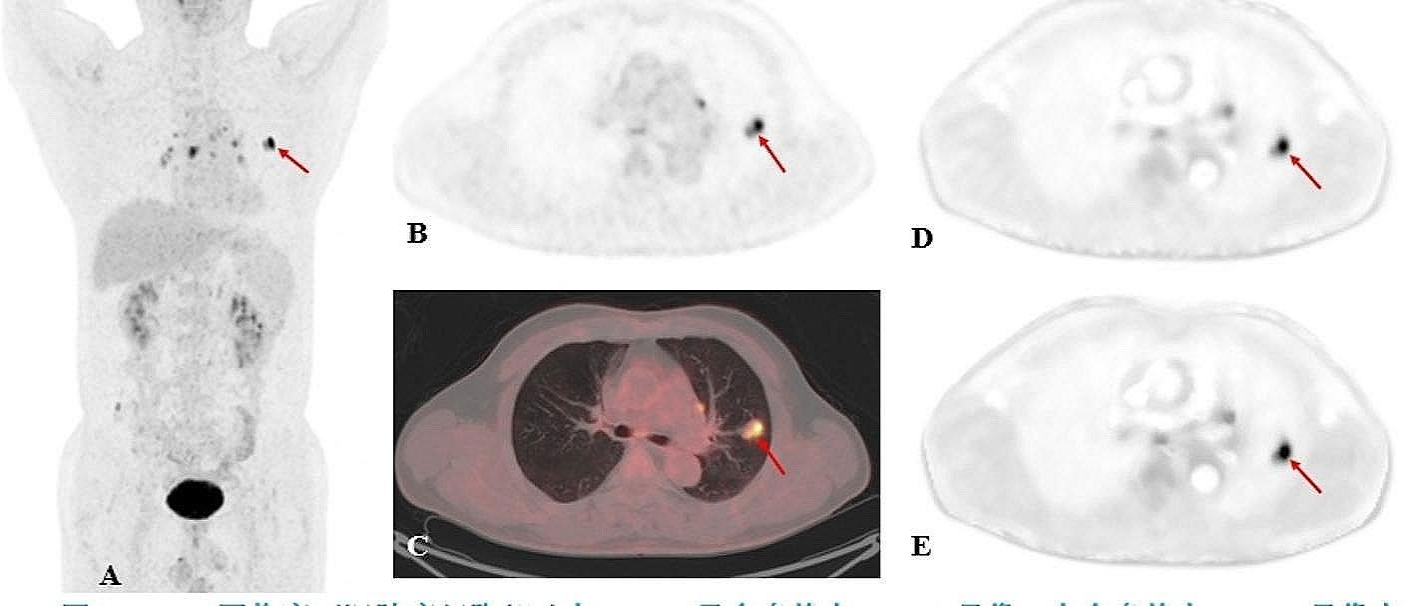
A 60-year-old male patient with clinical suspicion of lung cancer. PET/CT scan showed FDG-avid pulmonary nodules in the upper lobe of the left lung(red arrow), with a size of 2.2 × 1.3 cm, SUVmax of 12.2, Ki-65 min of 0.016 ml/g/min, and Ki-30 min of 0.014 ml/g/min. Surgical pathology confirmed a granulomatous lesion. In addition, PET/CT scan showed many FDG-avid LNs in the mediastinum. (A, static PET maximum intensity projection; B, static PET SUV image; C, static PET/CT fusion image; D, dynamic PET Ki-65 min image; E, dynamic PET Ki-30 min image)
